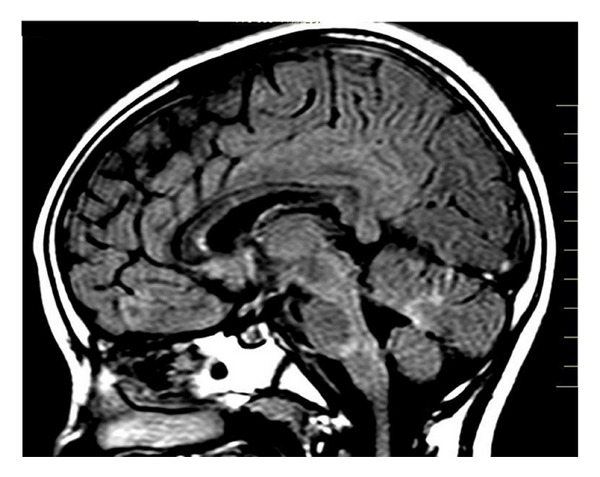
Brain MRI showing multiple infratentorial lesions.
